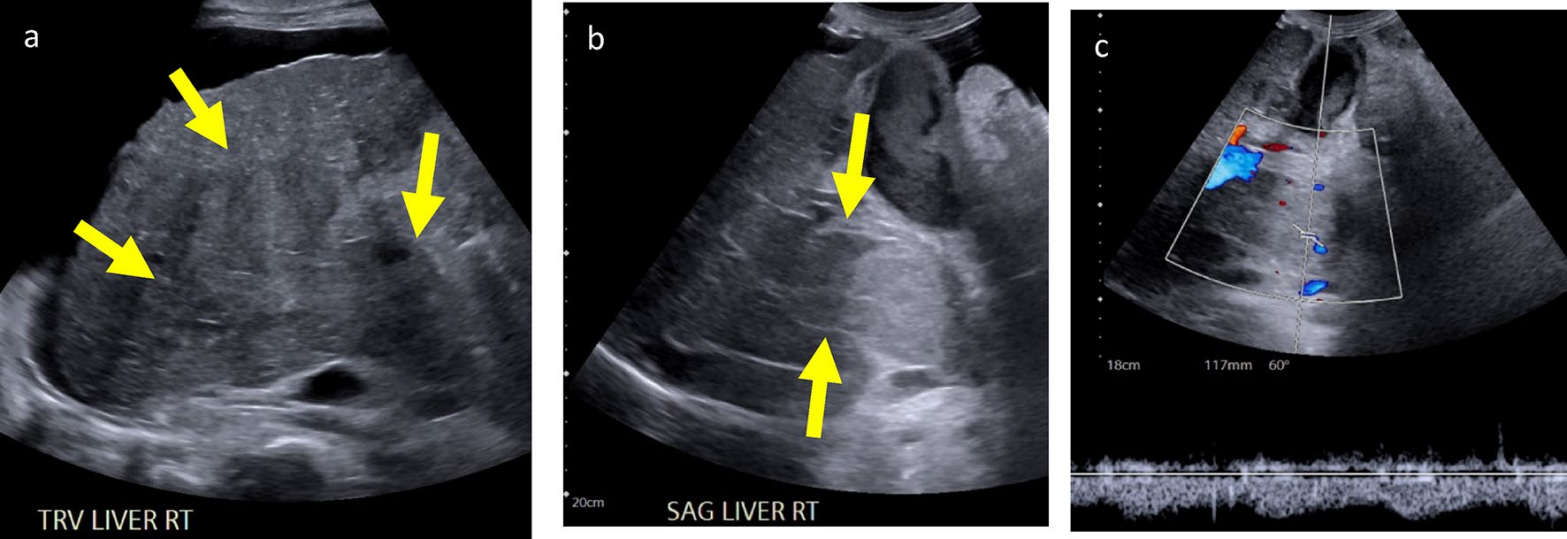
Infiltrative HCC and tumor in vein in a 62-year-old male with acute liver failure, presenting for urgent liver transplant evaluation. a Transverse grayscale US image shows a large area of parenchymal distortion in the right lobe of the liver (arrows), initially attributed to cirrhosis. The interpreting radiologist recognized the lack of a normal portal vein and requested additional images. b Sagittal grayscale US image of the porta hepatis using a larger field of view and the gallbladder (G) (filled with sludge) as a window, shows an expanded, thrombosed portal vein (arrows) with echogenic walls. c. Spectral Doppler image of the portal vein shows low resistance arterial flow diagnostic of tumor in vein
